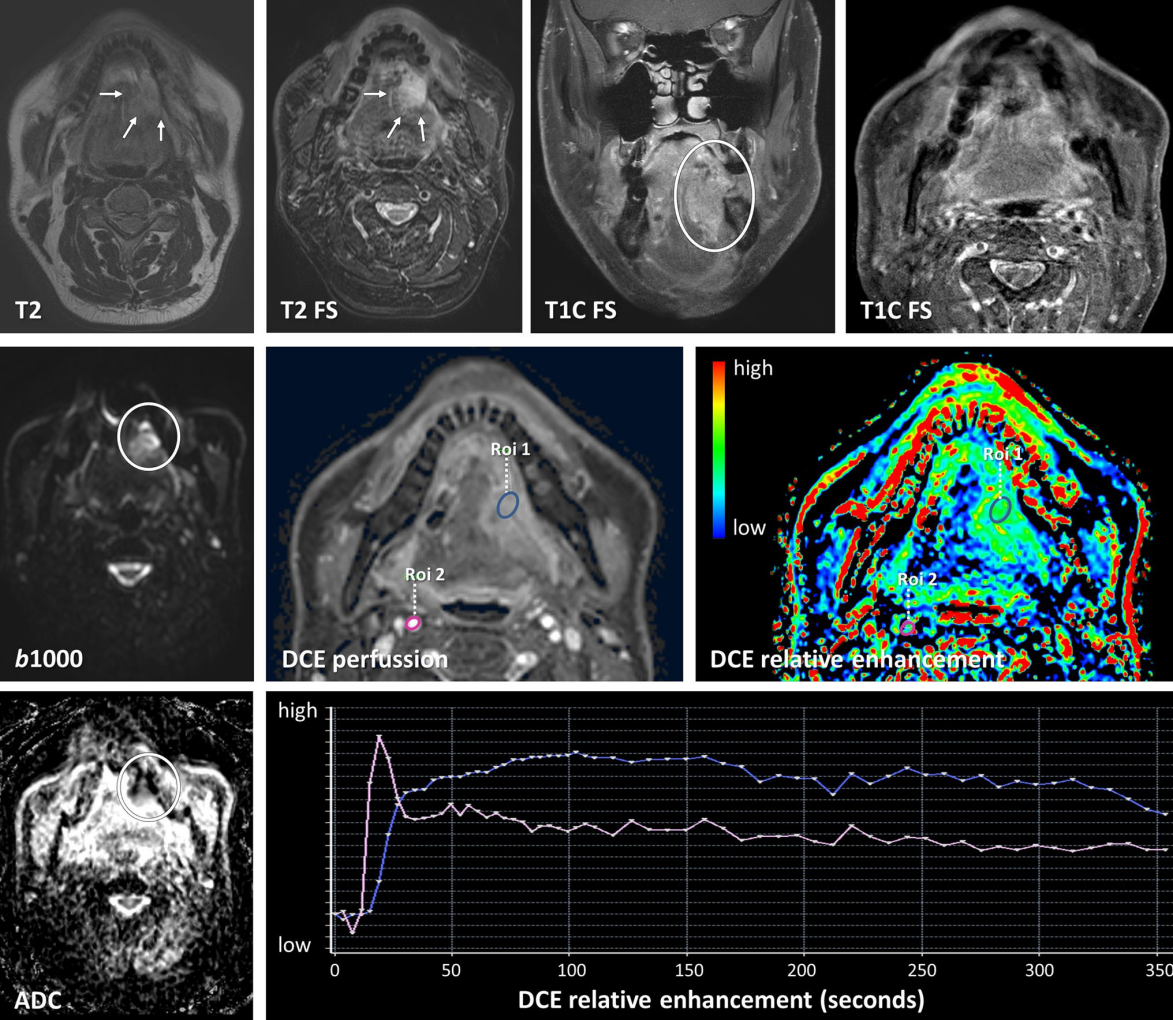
Tumor recurrence differentiated using diffusion and perfusion. A 57-year-old patient with a total resection of a pT2N0Mx lateral tongue carcinoma. Because of small free resection margins, a second resection was performed 1 month later with a submandibulectomy and free radial forearm flap reconstruction. Anatomical MRI showed changes during follow-up 6 months after resection with high signal on T2 with and without fat suppression. There is enhancement post gadolinium. Anatomical MRI was difficult to interpret as these findings could be due to both tumor recurrence as well as inflammation. Functional MRI demonstrated findings in keeping with tumor recurrence. Diffusion restriction was shown with high *b*1000 and low ADC values. Perfusion demonstrated increased AUC. Relative enhancement of the tumor (blue) showed a wash-in comparable to the carotid artery (purple) with plateau phase indicative for tumor. Tumor recurrence was pathologically confirmed (Color figure online)
